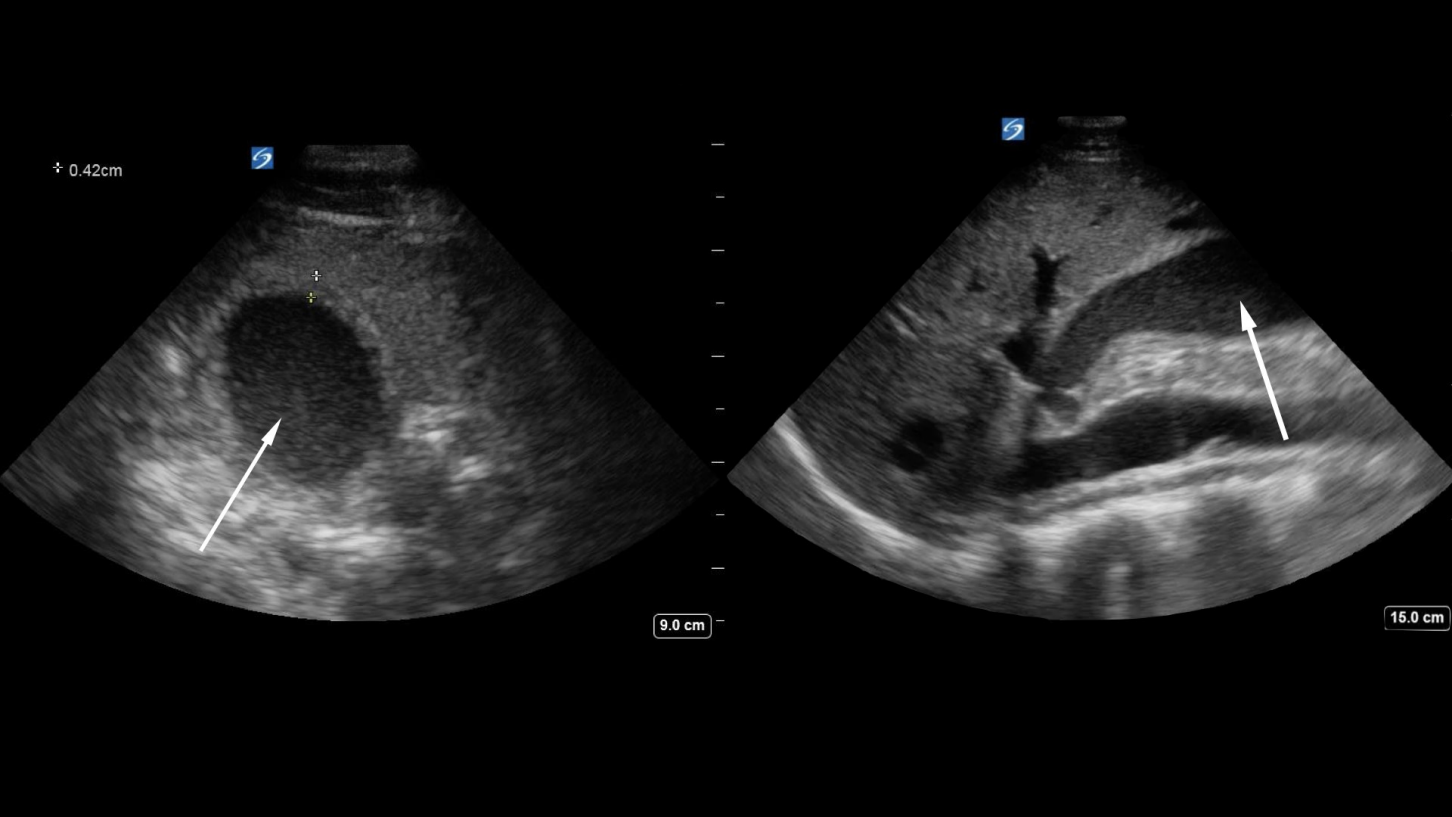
Left Pane: Ultrasound short axis view of gallbladder. Gallbladder is distended with sludge material within (Arrow). Anterior wall of gallbladder measured at 0.42cm (Calipers). Right Pane: Ultrasound long axis view of gallbladder. Gallbladder is distended with sludge material within (Arrow). No gallstones noted.
